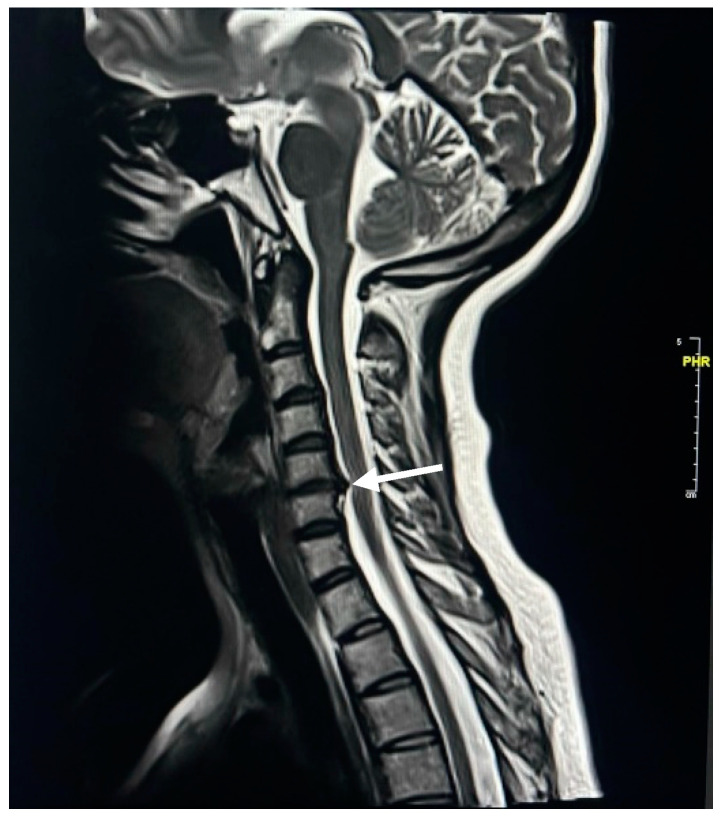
T2W MR image of disc herniation. The white arrow demonstrates disc herniation in the 5th intervertebral disc of a 46-year-old female with MS.
